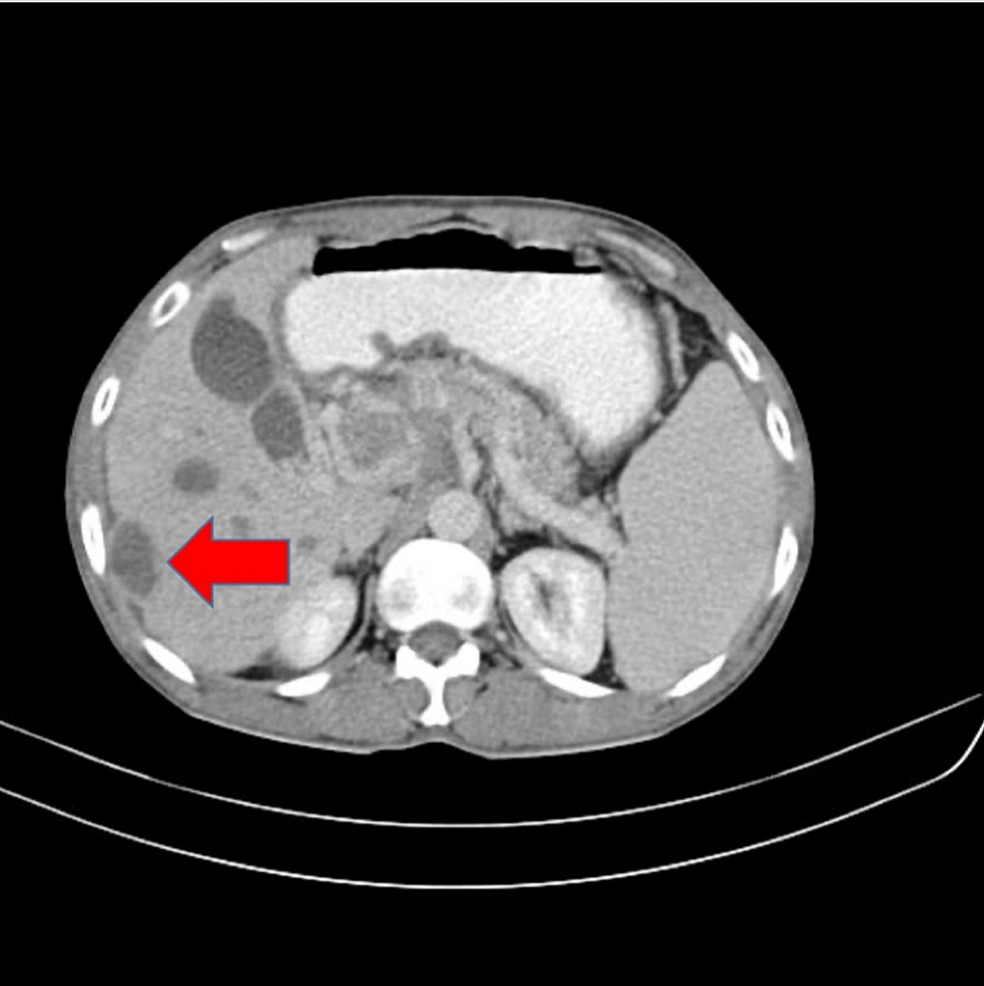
Contrast-enhanced computed tomography of the abdomen showing right subcapsular hepatic abscess
